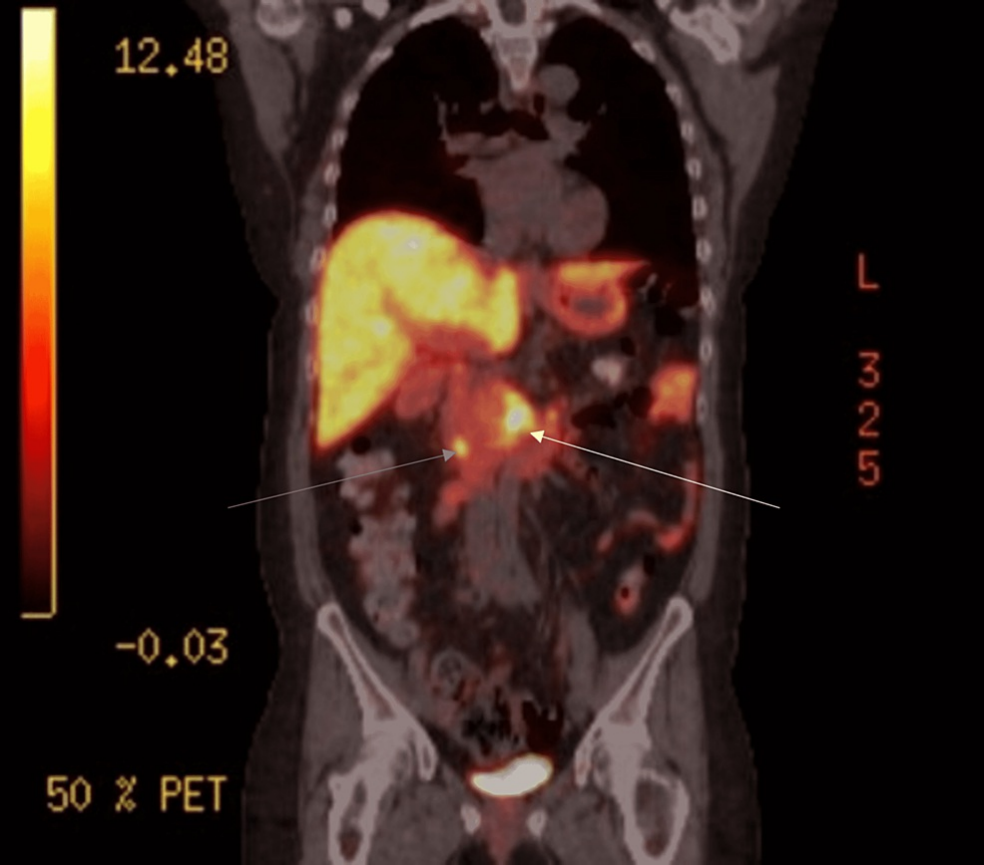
Coronal view PET/CT with hyperintensity in the second and third portions of the duodenum (blue arrow) as well as in the uncinate process of the pancreas (white arrow).
